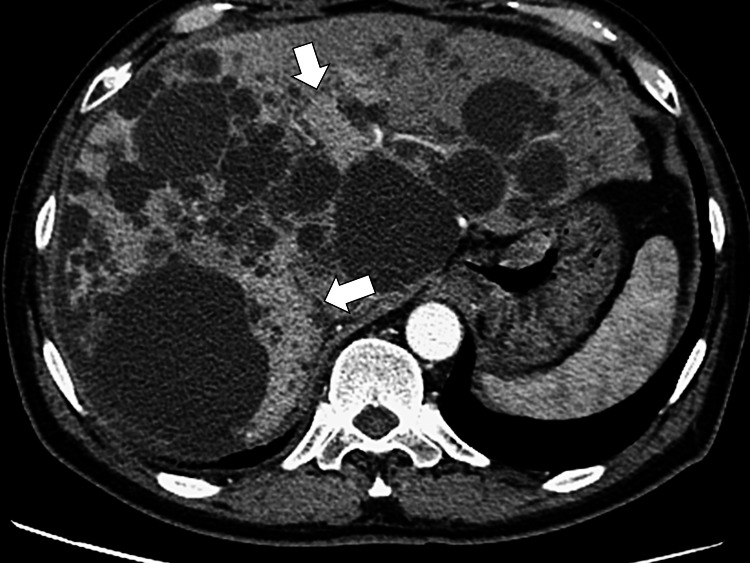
Enhanced computed tomography of the liver on admission. Enhanced areas around specific cysts indicate inflammation.
